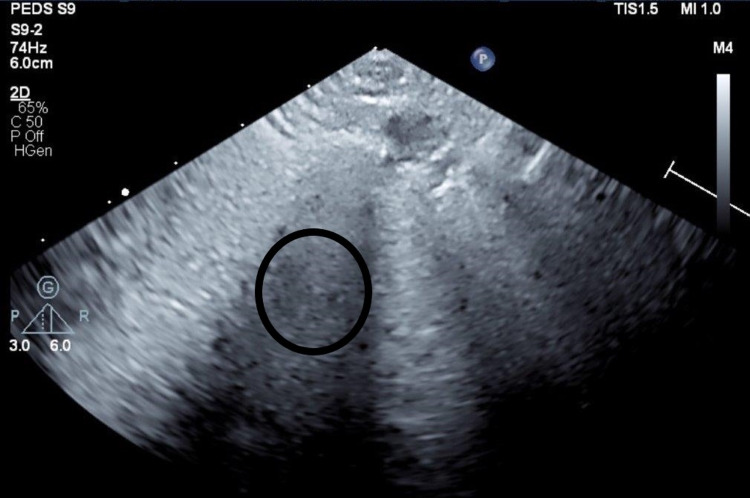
Lung ultrasound revealing obliteration of A-lines, confluence of B-lines (bright “comets”), and pulmonary hepatization (circled).
